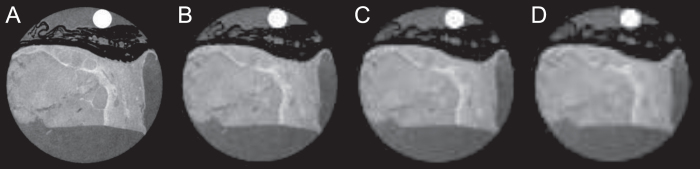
Influence of the image resolution on the gbPC-CT imaging results. GbPC-CT slice of a papillary renal cell carcinoma sample from Fig. 3 shown at different effective resolutions: (A) 100 × 100 μm^2^, (B) 300 × 300 μm^2^, (C) 400 × 400 μm^2^, (D) 500 × 500 μm^2^. Different effective resolutions were obtained through retrospective binning of the original data.
